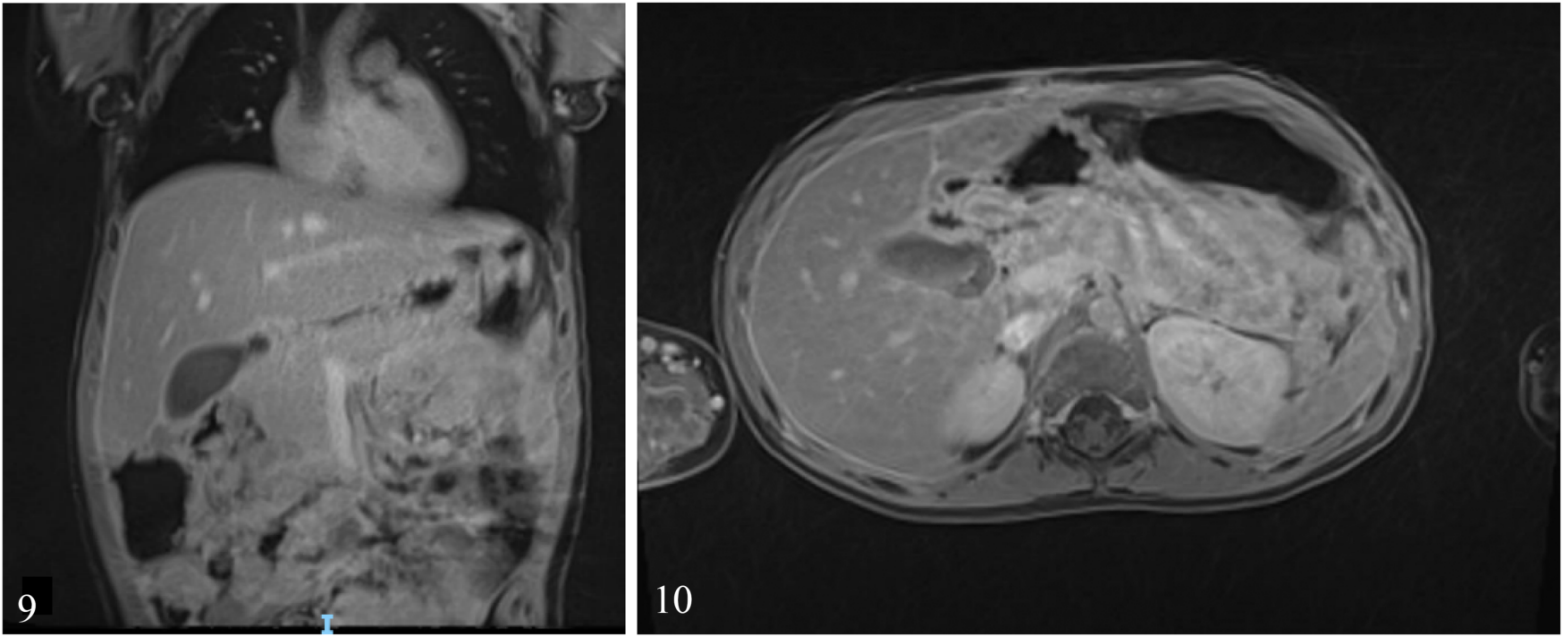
MRI scan showing no residual DDC or PPC 3 months after surgery.
